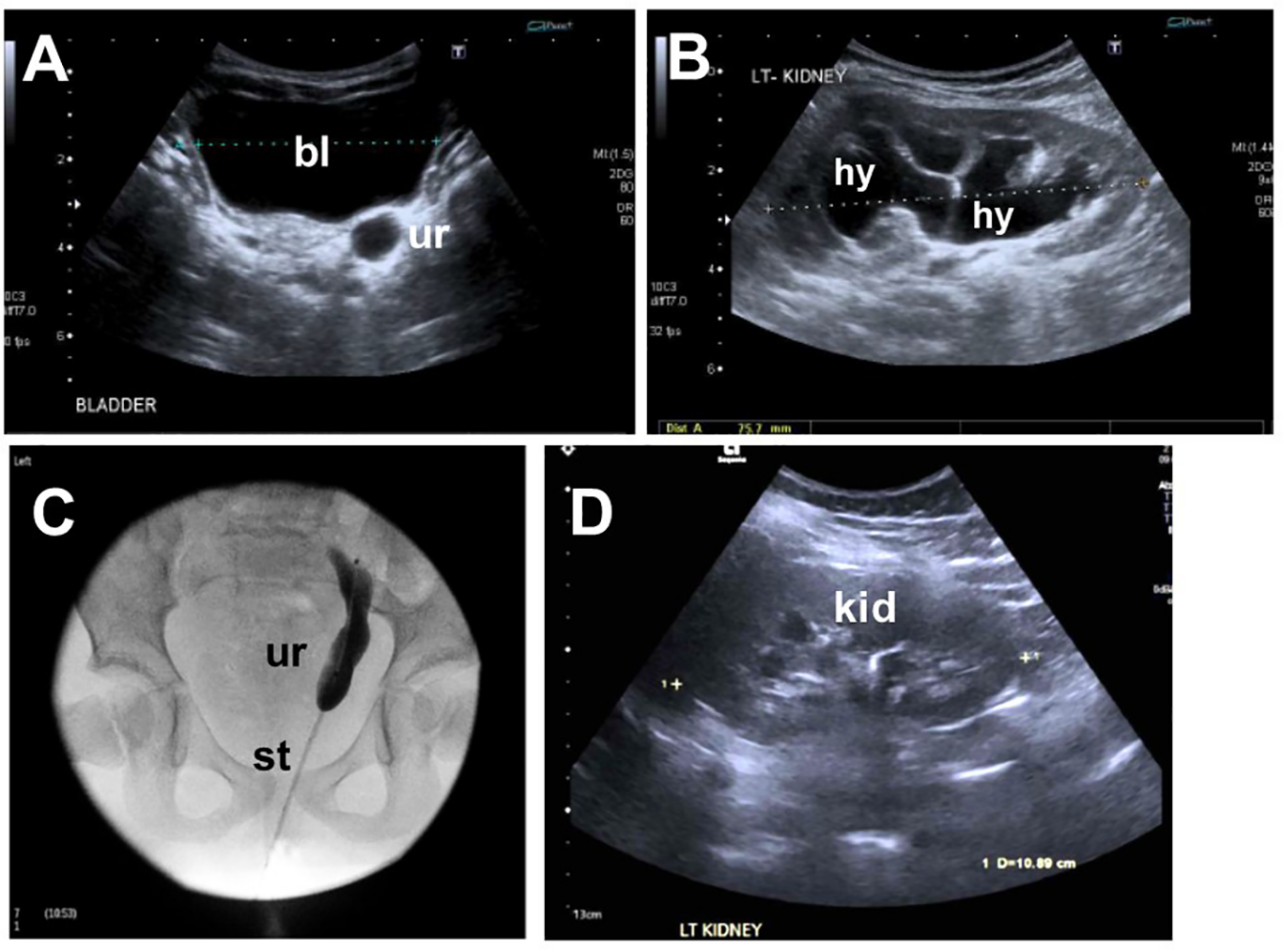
Imaging the lower urinary tract of the index case. (A) Ultrasound at 1 year of age showing a dilated distal ureter (ur) behind the bladder (bl). (B) Ultrasound at 1 year of age showing hydronephrosis (hy) of the left kidney. (C) Ureterogram at 4 years of age showing the stented megaureter. (D) Follow-up ultrasound shows resolution of hydronephrosis on the left kidney (kid).
